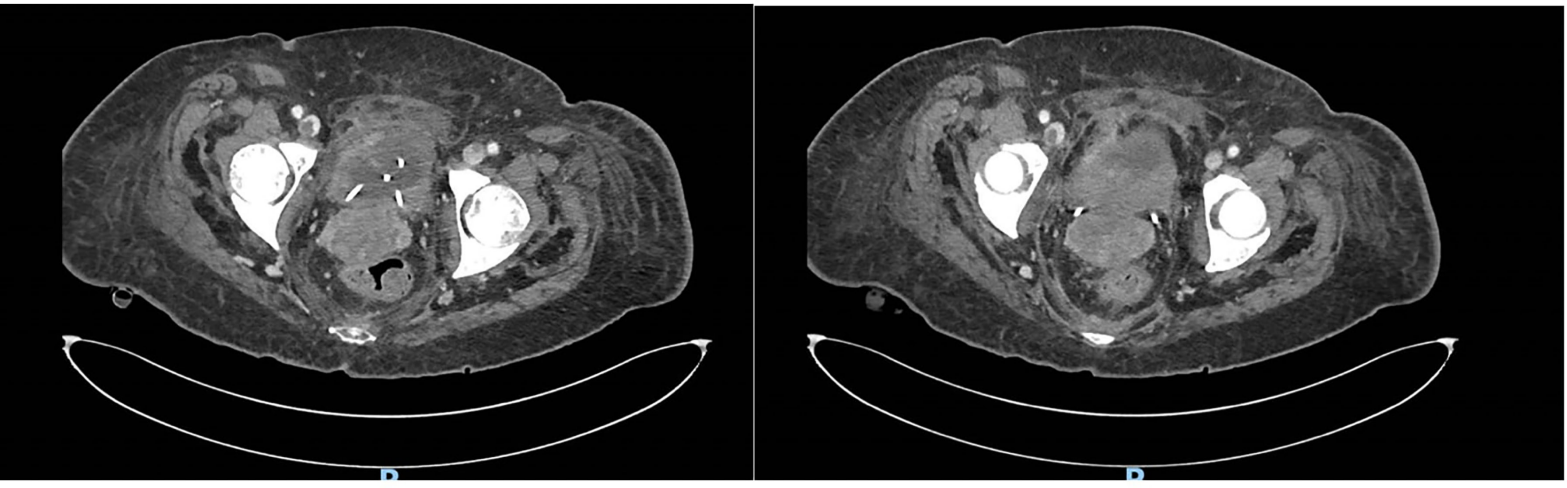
Bladder wall thickening documented on CT scan.
